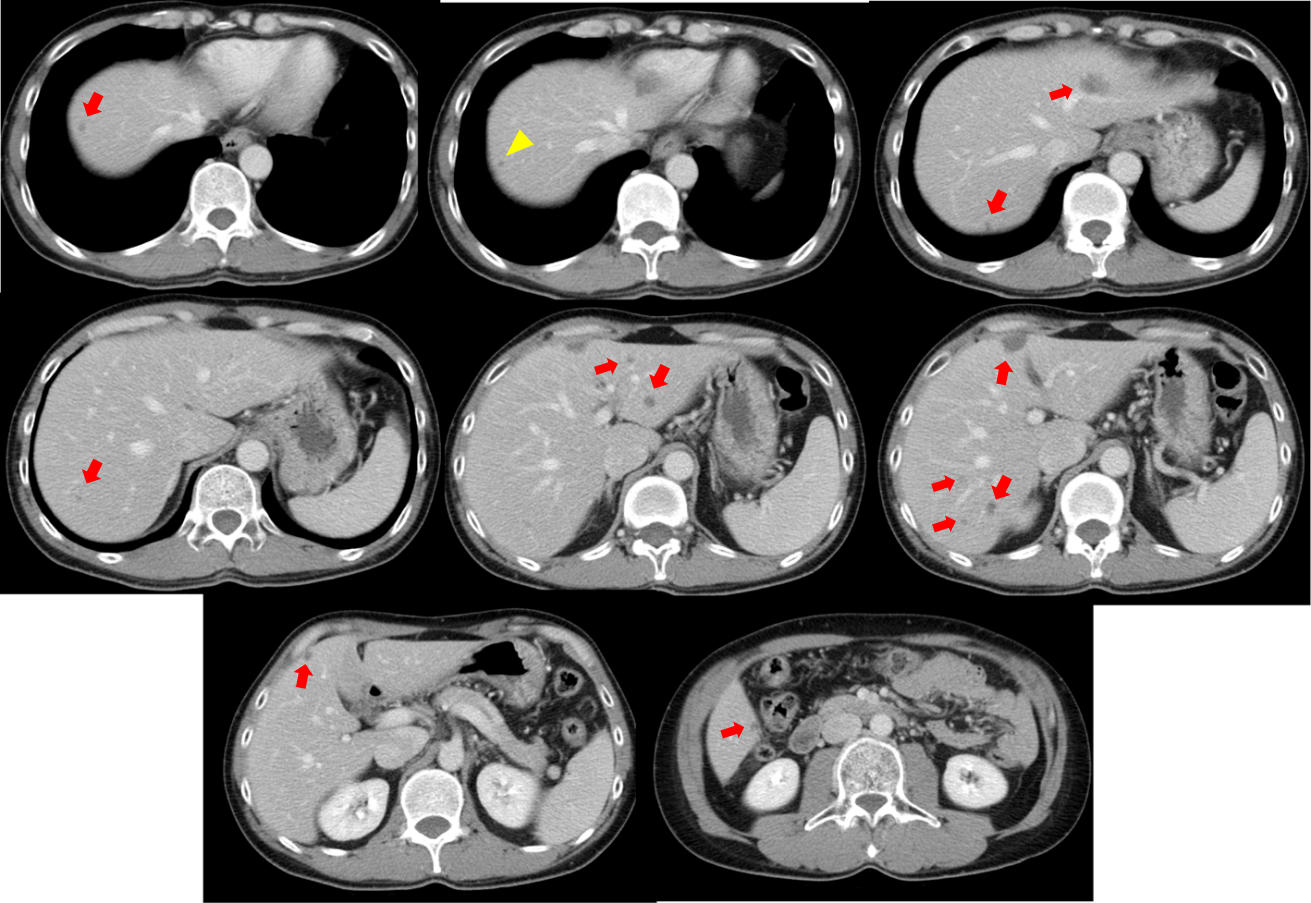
Contrast-enhanced computed tomographic image after 6 cycles of neoadjuvant chemotherapy. The 12 tumors detected before chemotherapy have decreased in size and have had an optimal morphological response. However, a new lesion has emerged in segment VIII of the liver (*arrowhead*)
